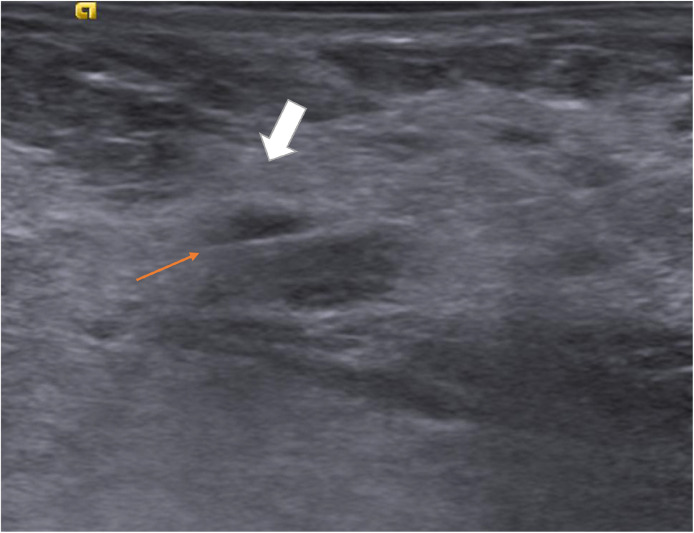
Ultrasound images obtained at the time of biopsy confirmed adequate sampling of the small right breast mass.
